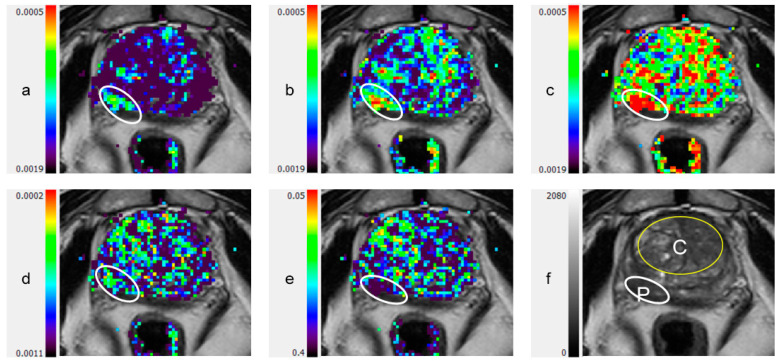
Peripheral zone lesion on color coded DTI Maps and T2-weighted image: (a) λ_1_; (b) λ_2_; (c) λ_3_; (d) maximal anisotropy (MA); (e) fractional anisotropy (FA); (f) T2-weighted image. C: central gland (transitional zone and central zone); DTI: diffusion tensor imaging; P: peripheral zone; White ellipsoid in (a) to (e): peripheral zone lesion.
